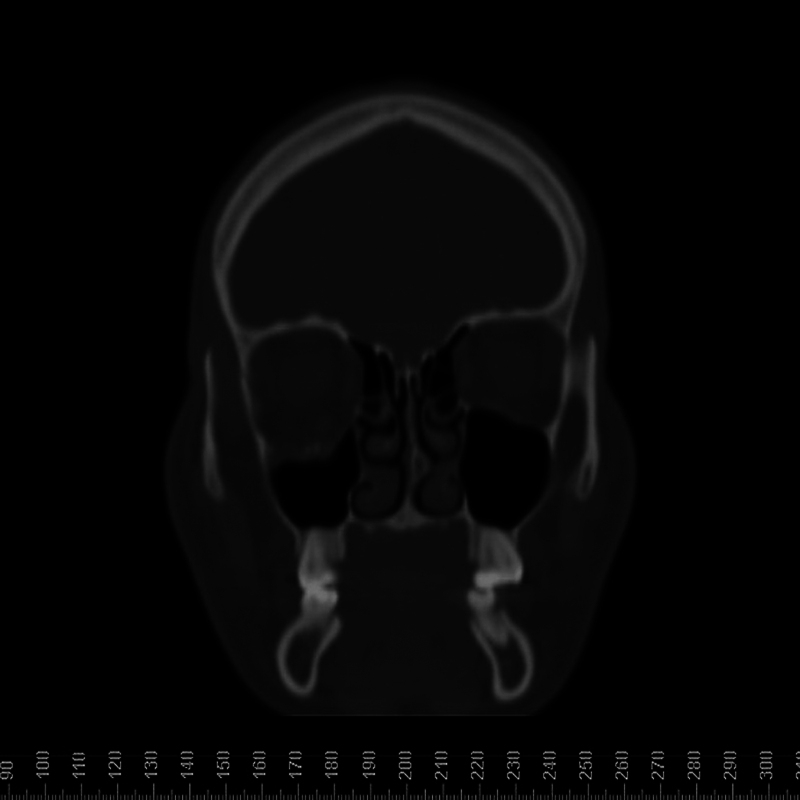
Computed tomographic (CT) scan of coronal anterior view.
